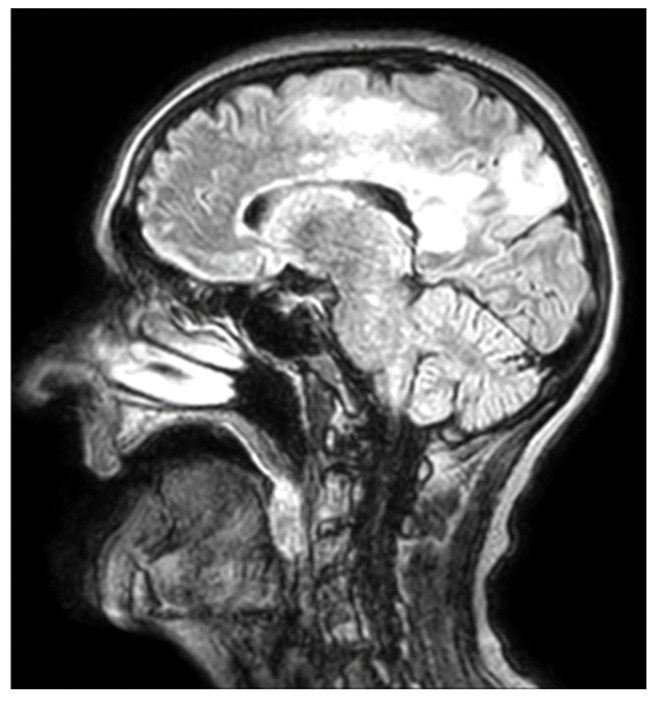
Sagittal FLAIR brain MRI showing a hyperintense lesion involving the left parieto-occipital white matter and extending toward the splenium of the corpus callosum, without mass effect or enhancement, which are findings consistent with progressive multifocal leukoencephalopathy.
